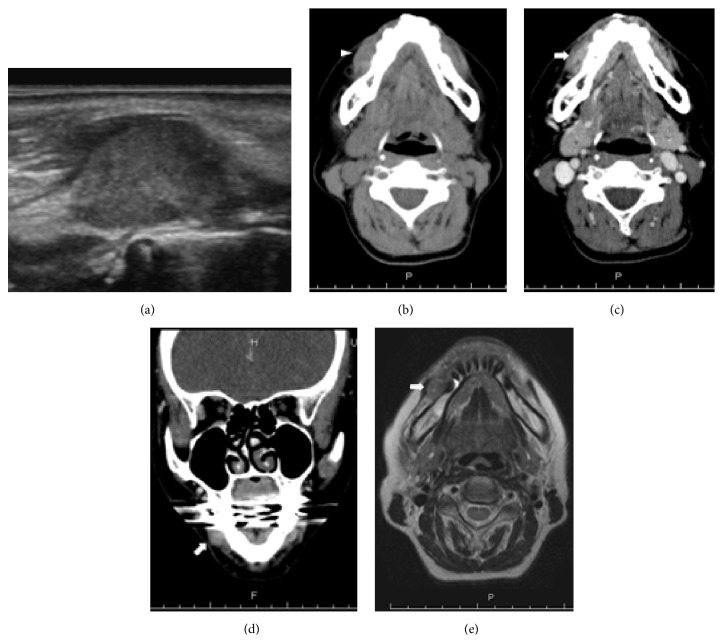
Findings of image examinations of the neck. (a) Echo showed a homogeneous hypo- to isoechoic lesion with a smooth margin. (b) Plain CT, axial view. Homogeneous low-density lesion (arrowhead). (c) Contrast-enhanced CT, axial view. (d) Contrast-enhanced CT, coronal view. Faintly enhanced lesions (arrows) were noted. (e) MRI, T2-weighted image, axial view. MRI showed a homogeneous low- to isointense lesion with partially high intensity (arrows).
